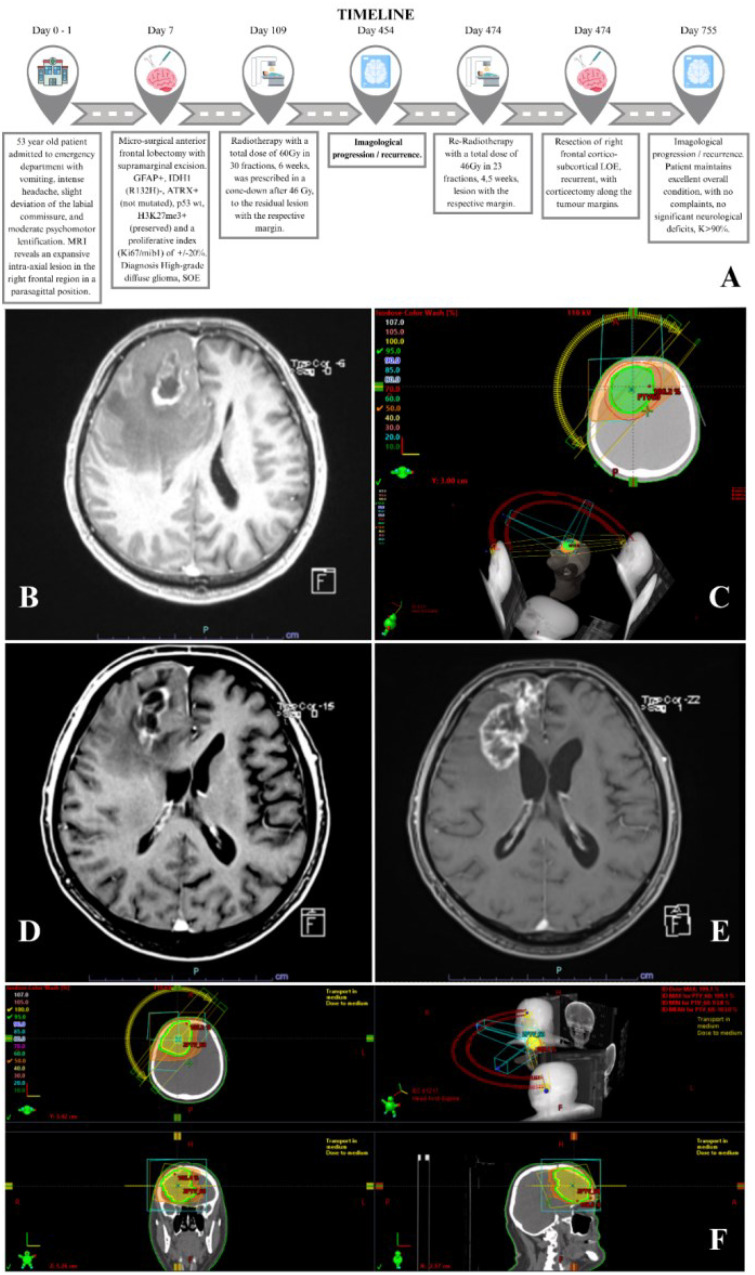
(A) Case report timeline. (B) Diagnostic MRI. (C) Radiotherapy isodose lines/beam configuration (initial treatment). (D) MRI showing pseudo-progression/necrosis. (E) MRI showing progression. (F) Radiotherapy isodose lines/beam configuration (re-irradiation).
